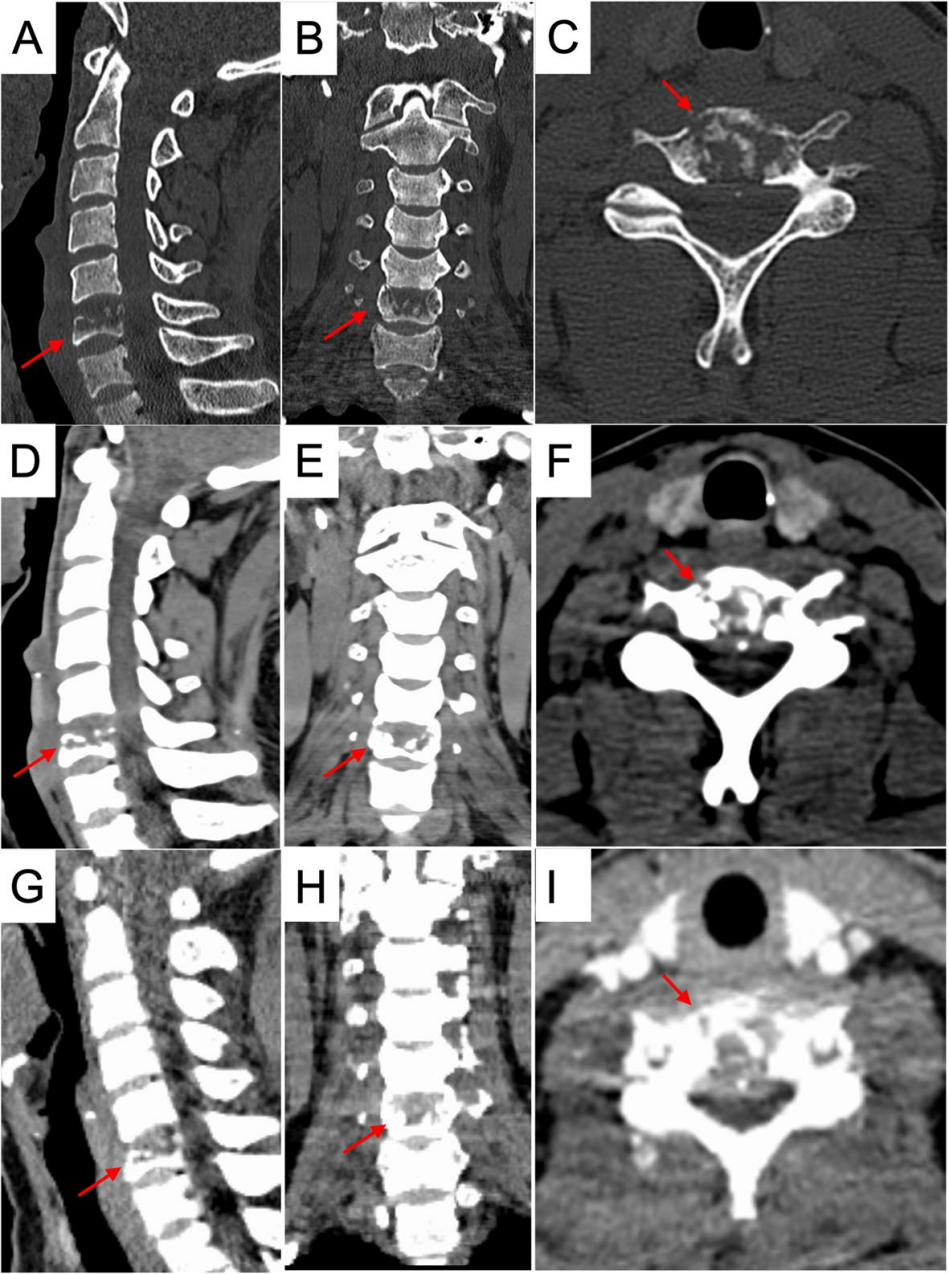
CT scan showing C6 vertebral body collapse and bone destruction. A to C bone window; D to F soft tissue window; G to I contrast-enhancement images; A, D, and G sagittal CT cuts; B, E, and H coronal CT cuts; C, F, and I axial CT cuts; arrows: C6 vertebral body
